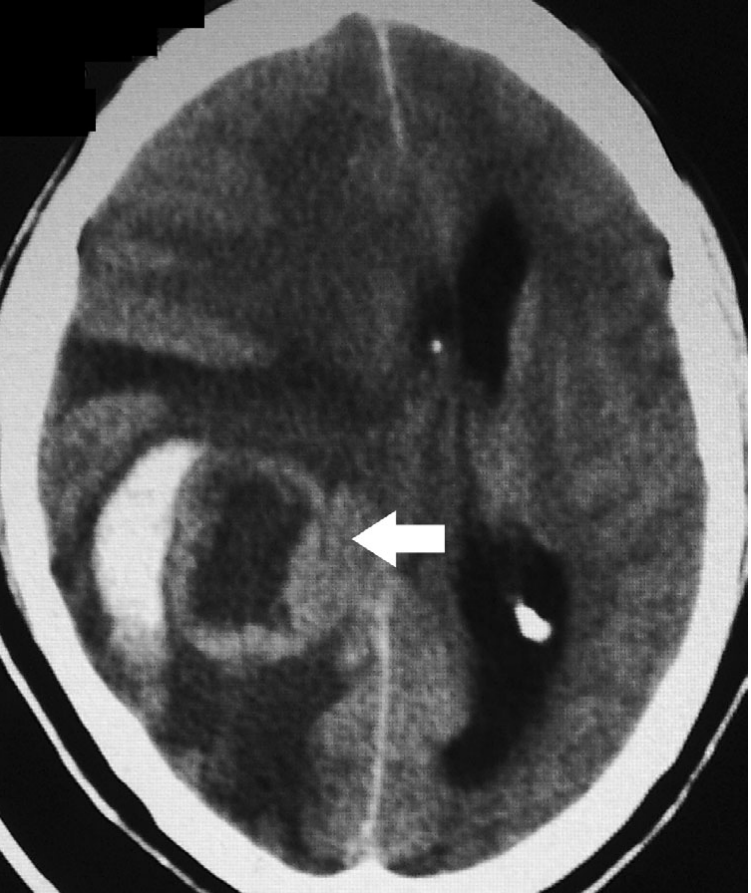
CT scan of the brain showing a 3.5 cm sized ring-enhancing mass (arrow) in the right parietal lobe with significant mass effect and compression of the right lateral ventricle. There is significant peri-lesional oedema.
